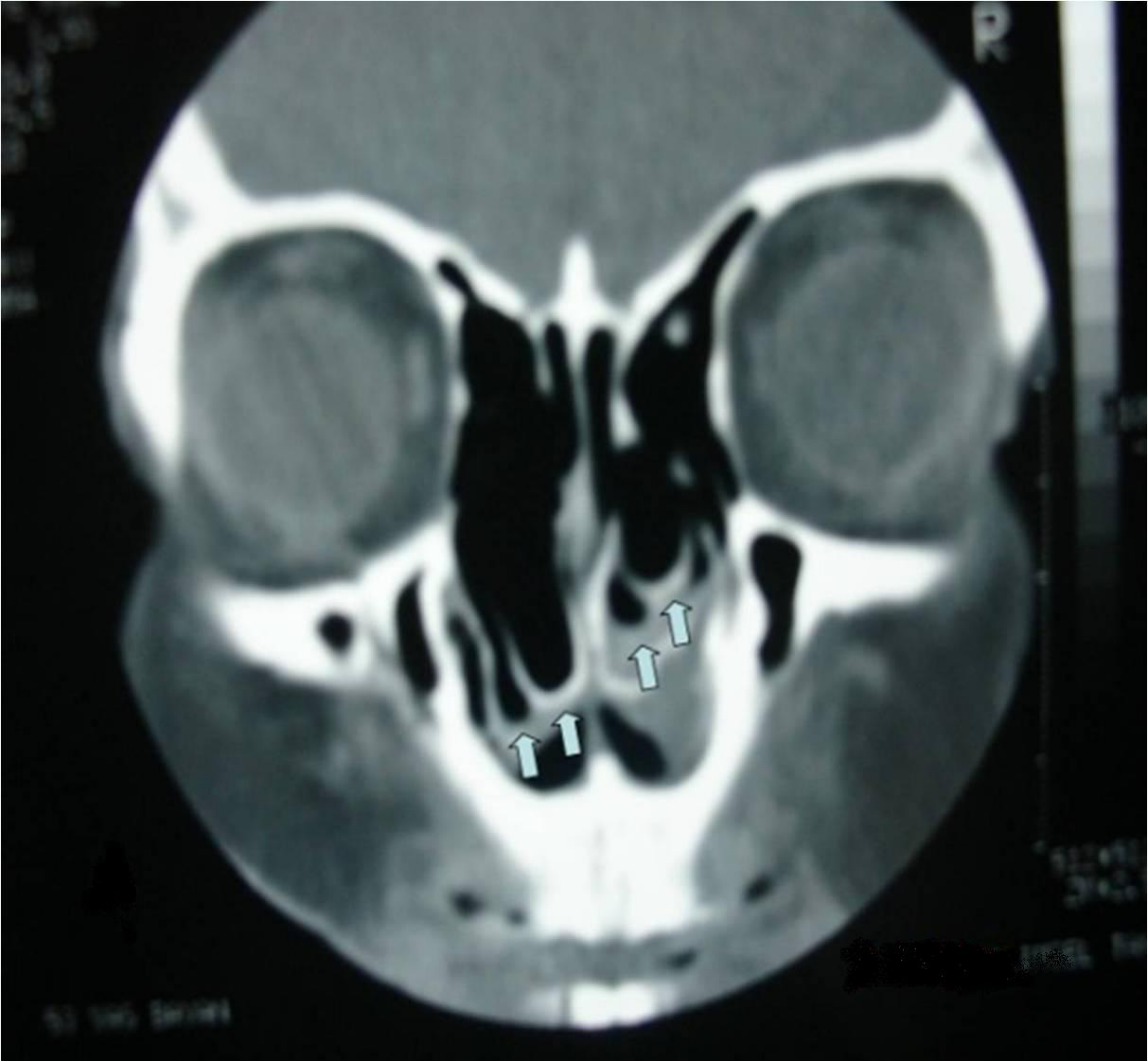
Coronal paranasal computed tomographic scan showing a concha bullosa inside the bilateral middle concha. Arrows indicate the inferior aspect of the middle conchae, from which the bilateral conchae bullosa originated.
